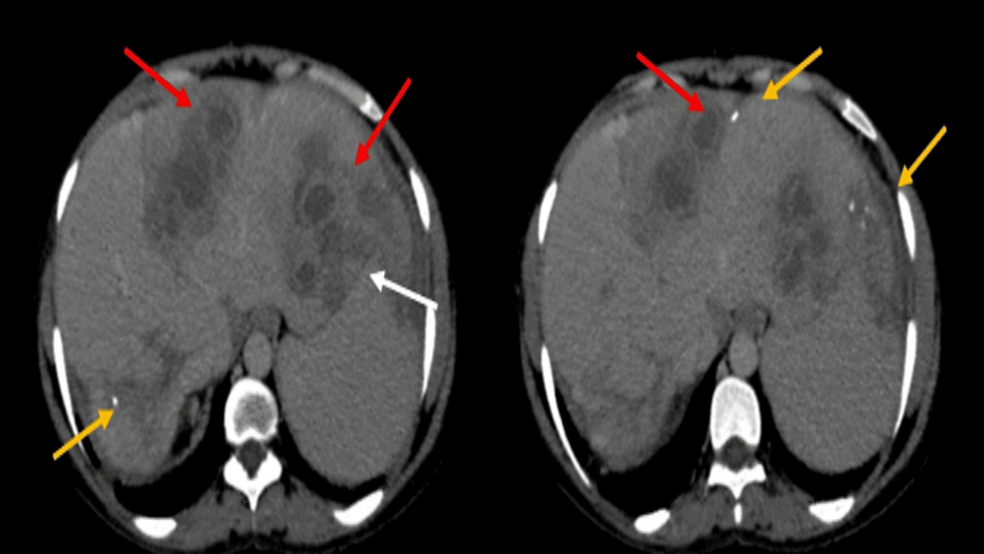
On contrast-enhanced CT, hypodense lesions are noted. Some give rim enhancement (target appearance) (red arrows), while others are not enhanced (white arrows). A few calcific foci are noted (yellow arrow).
